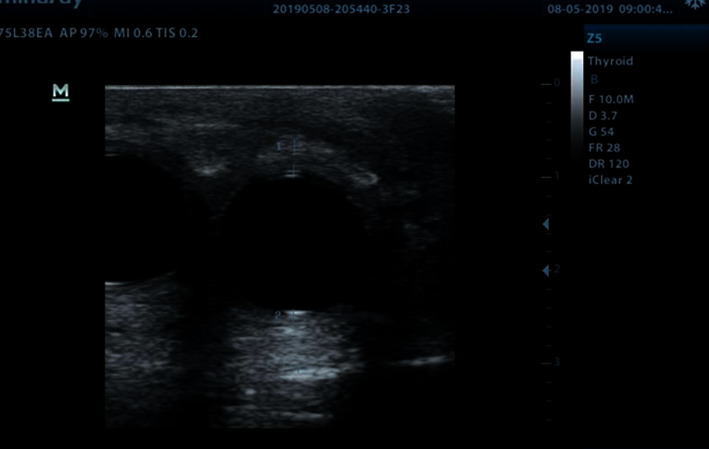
Inflated cylinders. 2D ultrasound image showing the inflated cylinders before penile hemodynamic measurement.
